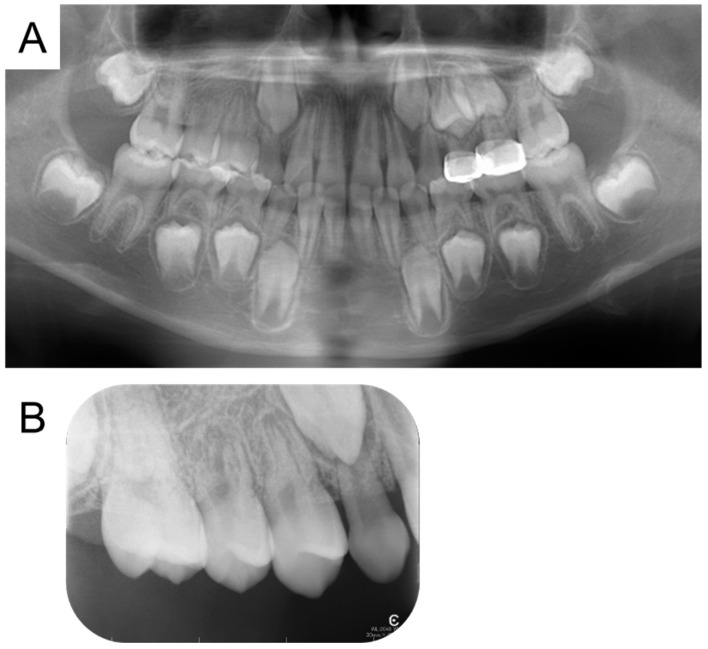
Radiographs at age 10 years: panoramic photograph (A), and periapical photograph (B).
